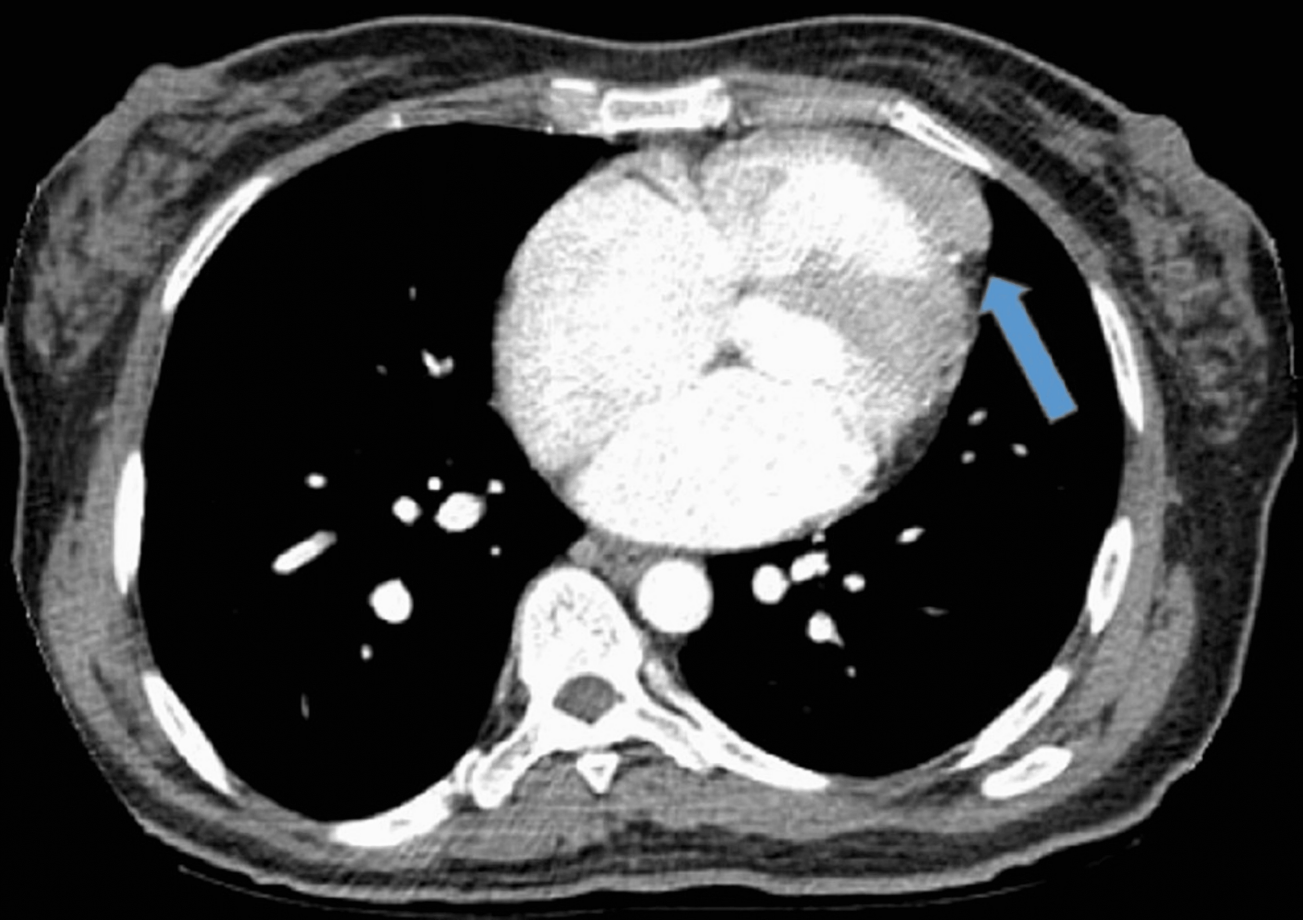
CT Chest With IV Contrast CT chest revealed a 3.6 cm ill-defined mass-like lesion noted in the right ventricle/pericardium; there was no contrast filling ventricular aneurysm, pericardial-based mass/metastasis, or an abnormal lymph node.
